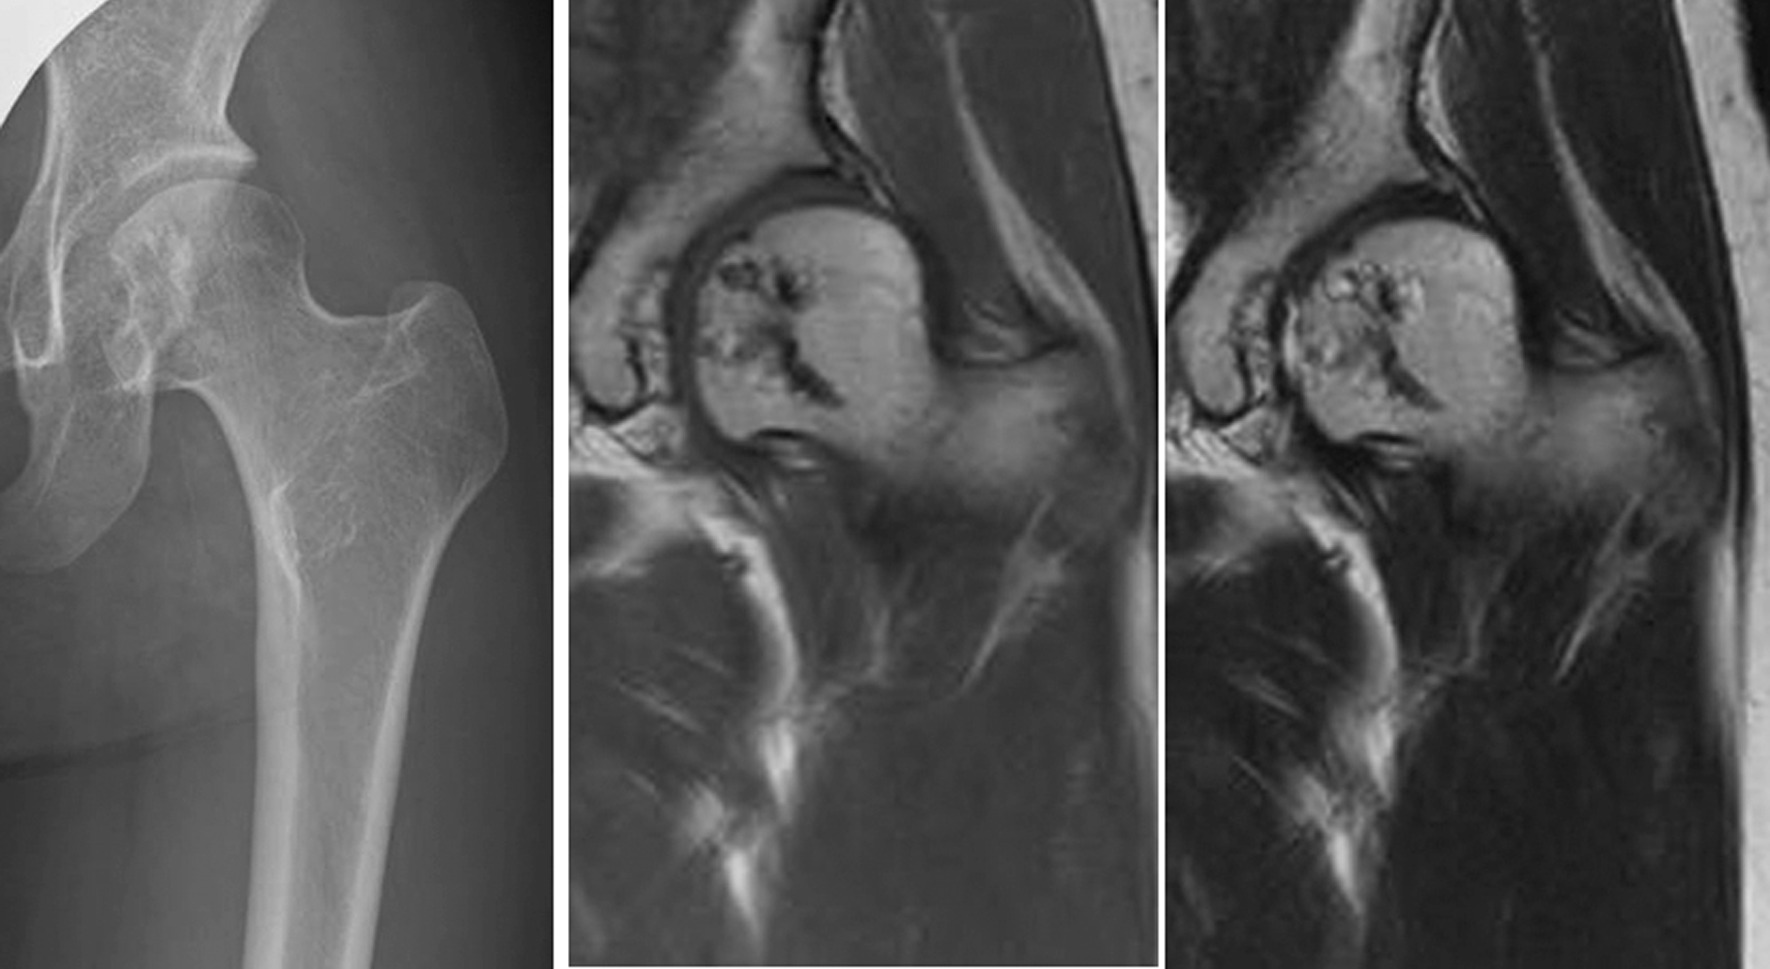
Imaging findings obtained 10 years postoperatively: Case 2. Plain X-ray image (left) and T1- and T2-weighted (middle and right, respectively) MRI images demonstrating that there is no recurrence or avascular necrosis
